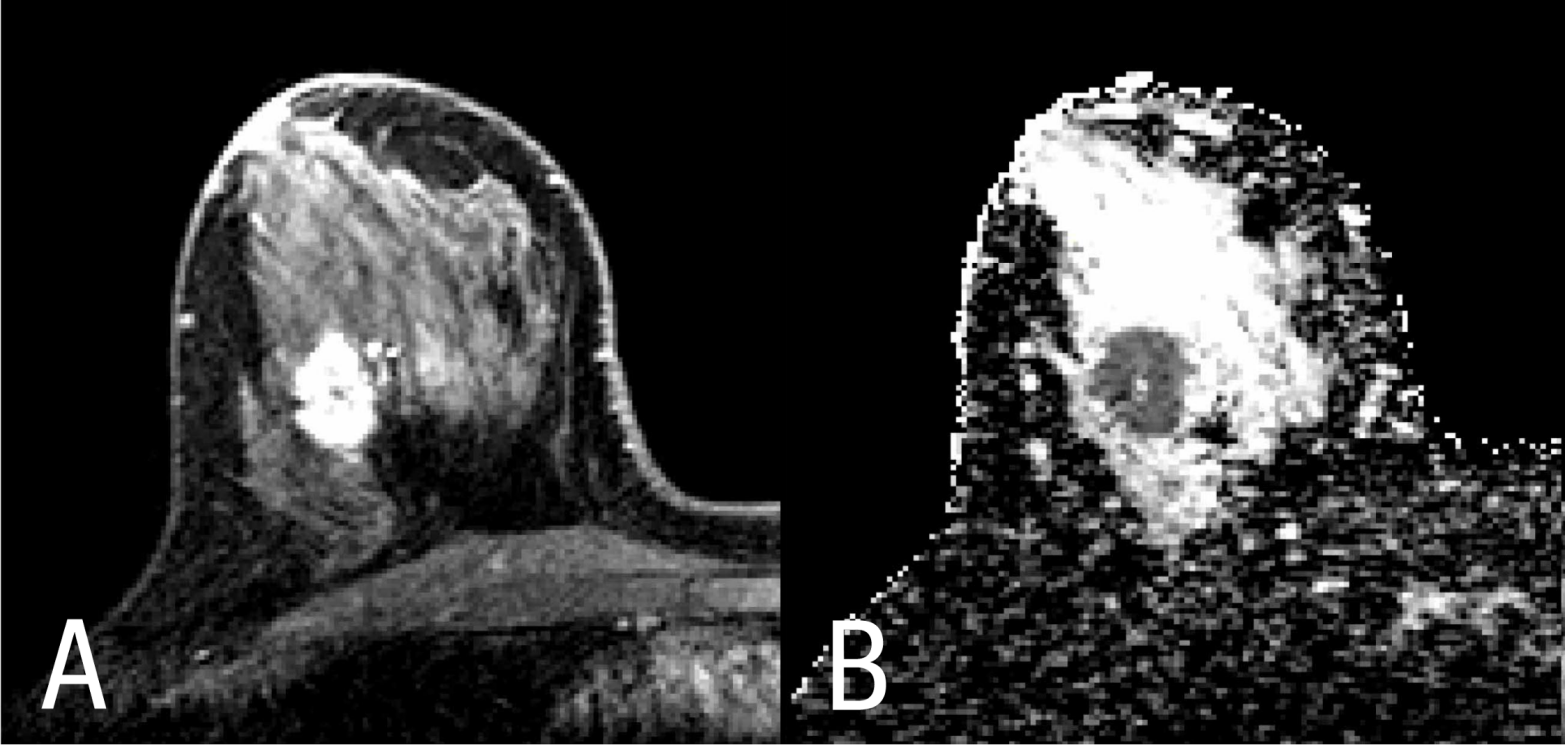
Lesion differentiation in (A) dynamic contrast-enhanced magnetic resonance imaging (MRI, left) and in (B) diffusion-weighted imaging (DWI, right) of the right breast of a 41-year-old woman.
